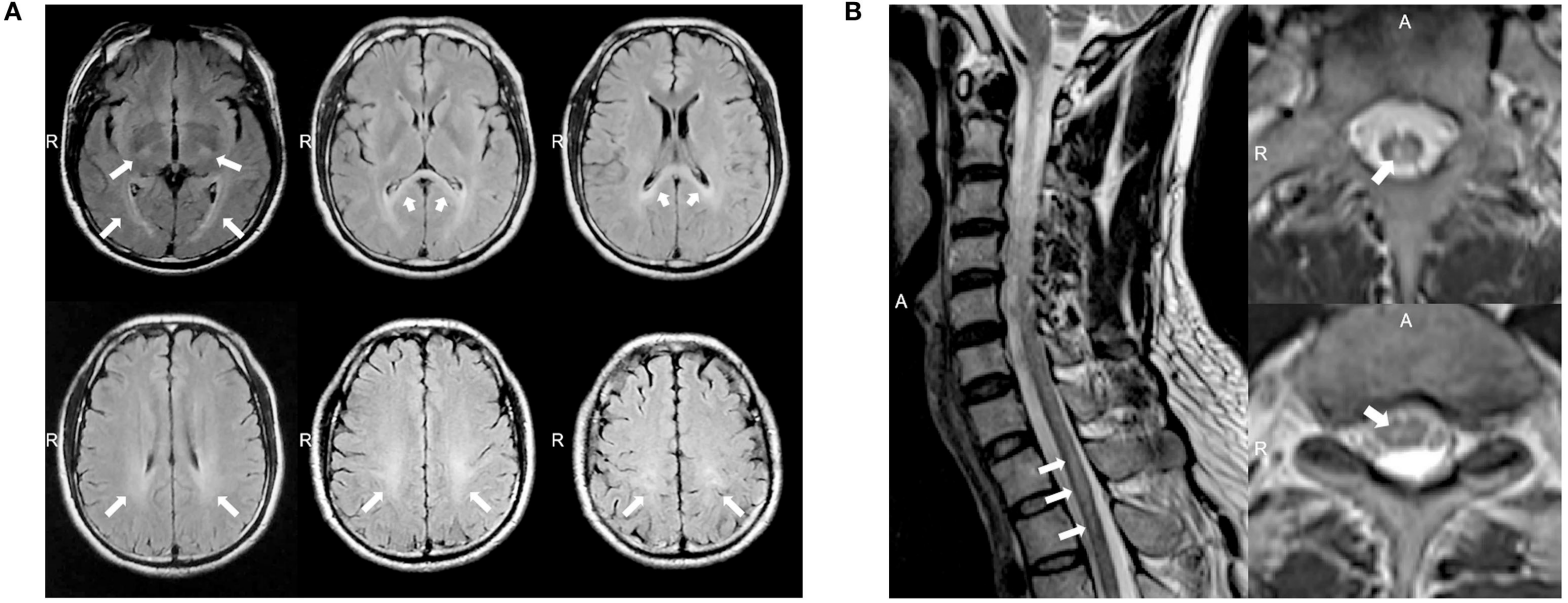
(A) Magnetic resonance imaging (MRI) of the brain for case 1 showed abnormal consecutive hyperintensities along the bilateral corticospinal tracts and in the optic radiation and corpus callosum on T2-weighted (T2W) fluid-attenuated inversion recovery (FLAIR) images (white arrowheads). (B) MRI of the spinal cord for case 1 showed abnormal intramedullary lesions from T1 to T2 on T2W images (white arrowheads).
